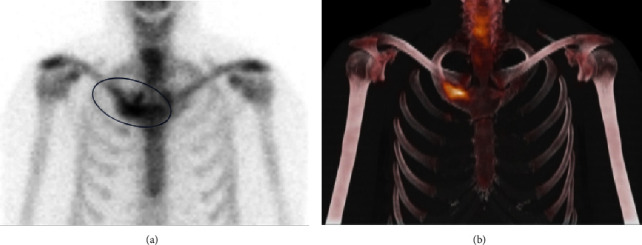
Bone scan with SPECT/CT imaging following technetium-99 m medronate injection (Tc-99 m MDP). Planar projection of the thorax (a) and 3D maximum intensity projection (MIP) CT + SPECT (b) show increased radiotracer uptake in the right first rib and costochondral junction corresponding with the enlarged bone.
